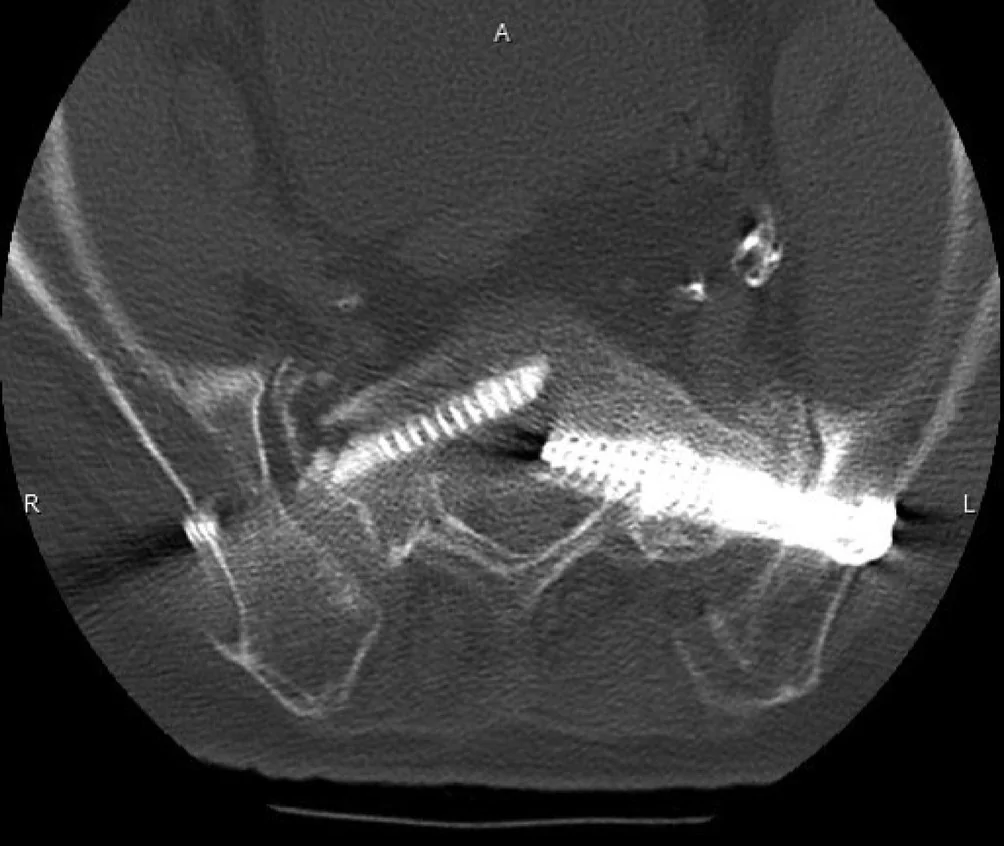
CT image showing the left SI screw partially contacts the cortical bone but does not violate the sacral foramen.
